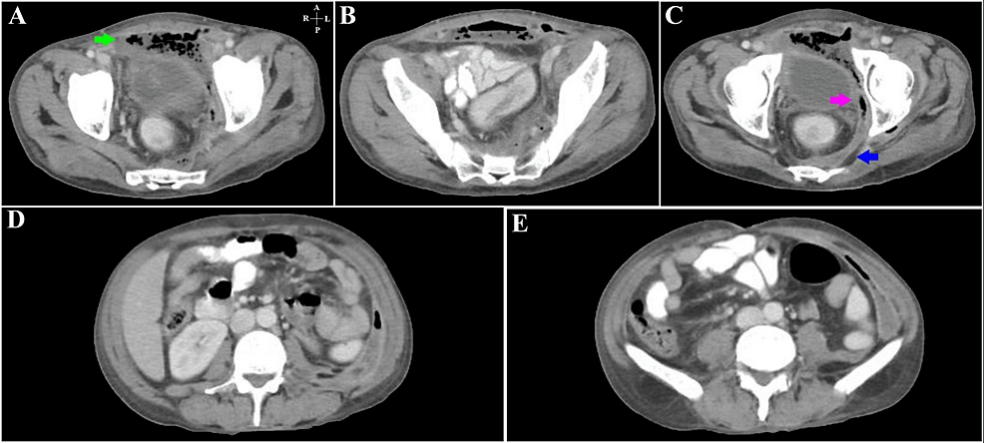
Patient 6 - Contrast-enhanced CT of the abdomen and pelvis in the axial plane Figure 8A – Axial images through the pelvis with a large air-containing collection (green arrow) seen anterior to the urinary bladder and along the left hemi-pelvic wall. A few air foci are also seen in the left rectus abdominis muscle. Figure 8B – A peripherally enhancing, air-containing collection is noted in the pre-peritoneal space posterior to the rectus abdominis muscle and the presacral space. Figure 8C – At the level of the femoral heads; a peripherally enhancing air-containing collection (pink arrow) is seen along the left hemi-pelvis extending anteriorly in the extra-peritoneal space. The collection (blue arrow) is also seen extending posterior to the rectum. Stranding of surrounding fat is noted. Figure 8D – L3-L4 level; An air-containing peripherally enhancing collection is seen along the left lateral abdominal wall with stranding of fat medially. Figure 8E – The peripherally enhancing collection is seen along the left abdominal wall medial to the transversus abdominis muscle with air within.
